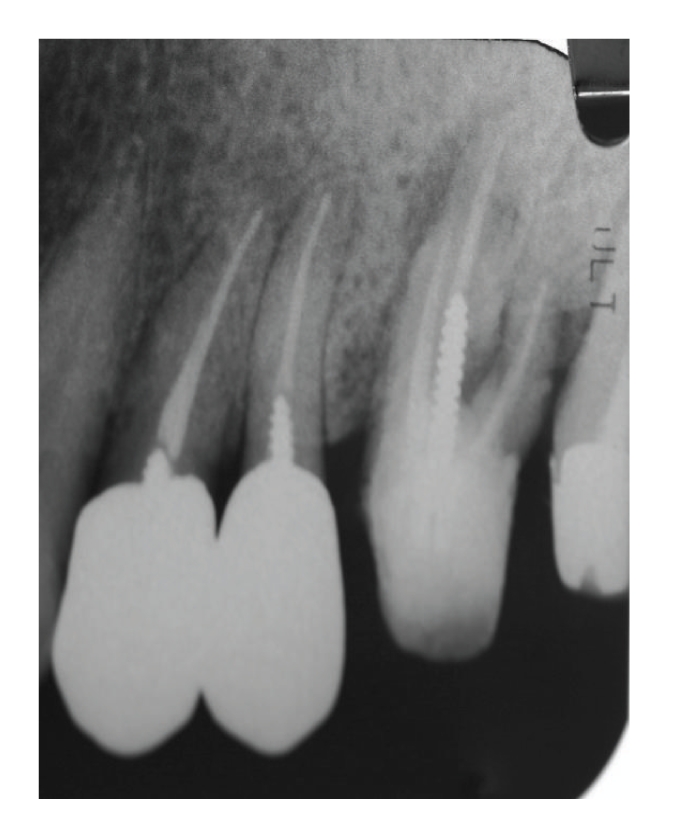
Case 1: Radiography 2 months after the initial visit showing that the radiolucency at the apex had decreased.
